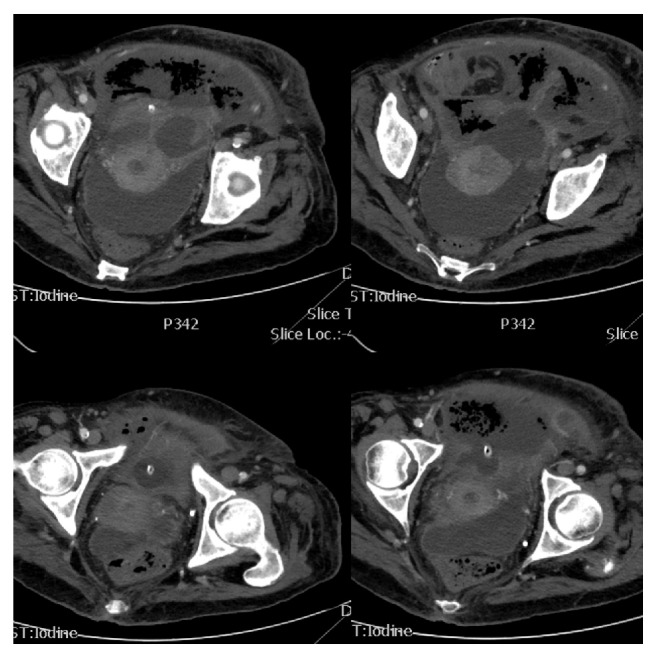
Abdominal CT scan images revealing the presence of gas within the anterior bladder wall and retropubic space. The anterior bladder wall is not enhanced by contrast material.
